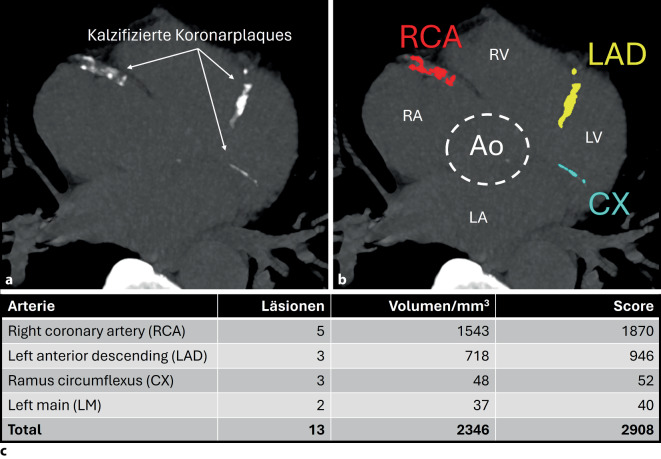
Kalziumscoring eines 74-jährigen Mannes. Multiplanare Rekonstruktionen einer nativen CT-Untersuchung veranschaulichen die kalzifizierten Koronarplaques in den Originalbildern (a) und die farbkodierte Kalkerkennung (b). *Rot *„Right coronary artery“ (*RCA*); *gelb *Ramus interventricularis anterior („Left anterior descending”, *LAD*); *türkis *Ramus circumflexus (*CX*). Der linke Hauptstamm („Left main“) ist in dieser CT-Schicht nicht miterfasst. c Automatische Quantifizierung und Zuordnung zu den Koronararterien; der Agatston-Score des Patienten beträgt 2908. *Ao* Aortenklappe, *LA* linkes Atrium, *LV* linker Ventrikel, *RA* rechtes Atrium, *RV* rechter Ventrikel
